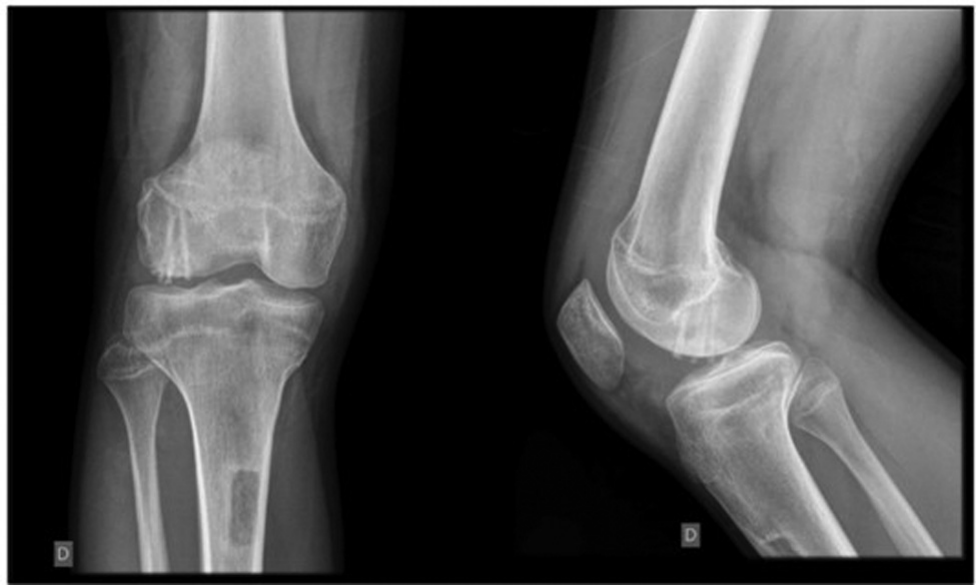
X-ray 4 months after the surgery.
